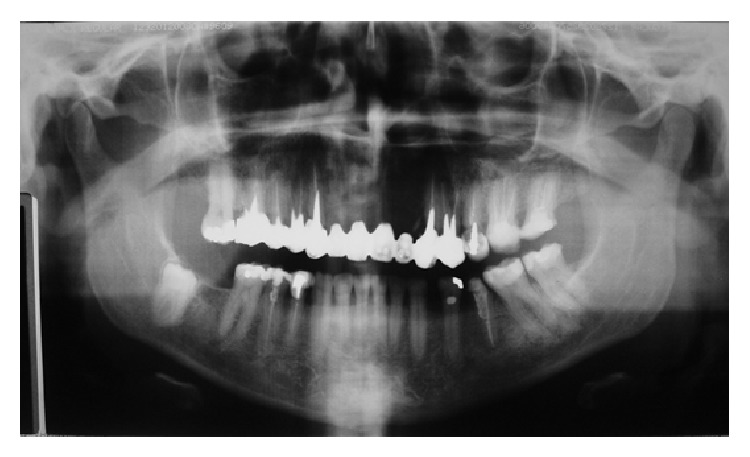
Findings of initial panoramic radiograph. Insufficient length of filling material of teeth 23, 24, 25, 26, 27, 15, 16, 45, 44, and 35. Old long fixed prosthesis included teeth 23, 24, 13, 14 15, and 16. Absent anterior teeth (22, 21, 11, and 12).
